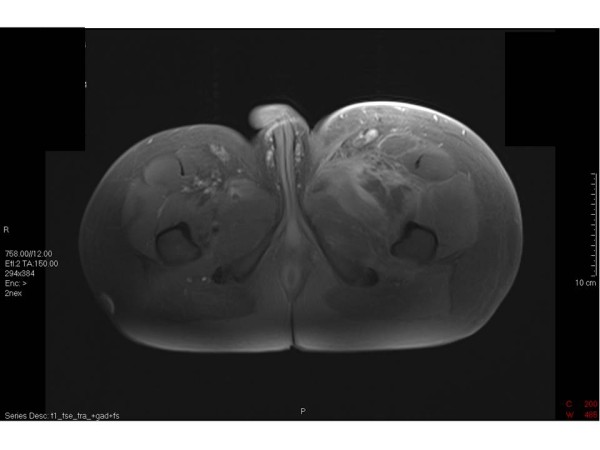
MRI Pelvis and Thighs. An area of decreased signal inferior and lateral to the left femoral neurovascular bundle. This was suggestive of tissue necrosis.
